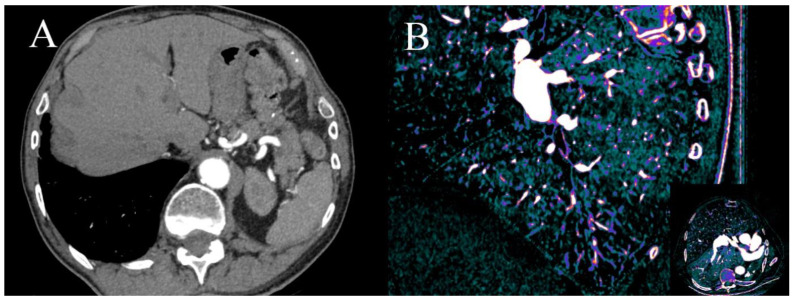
Dual-energy coronary CTA performed due to worsening of dyspnea and suspected CAD. There is small clot in peripheral artery in segment 9 of right lung which can be easily misted in axial VMI at 70 keV (A). There is a large V-shape aerial of hypoperfusion visible in sagittal reformat on iodine(water) map in segment 9 (B); similar aerials were discovered in segments: 4R and 5R. Finally, diagnosis of chronic peripheral pulmonary embolism was made.
